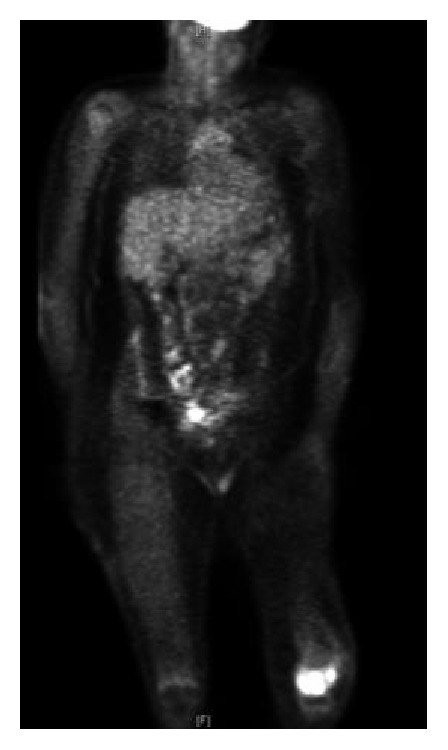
Fludeoxyglucose-positron emission tomography (FDG-PET), August 2009. Increased activity of FDG within the left knee (SUV max of 17.11), with physiologic distribution of FDG in the remainder of the body.
